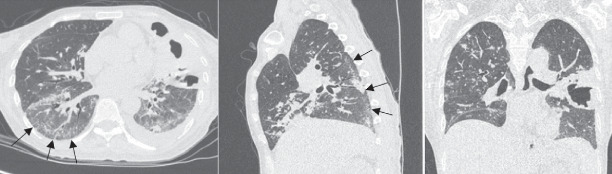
High-resolution CT scan of the chest showing reticular patterns in the posterobasal segments of both lower lobes with subpleural sparing, consistent with a NSIP pattern of ILD.
